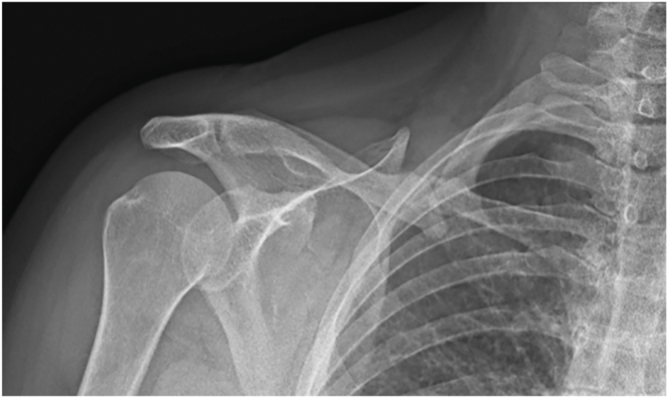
Frontal view radiograph showing a clavicular midshaft hypertrophic pseudarthrosis.
